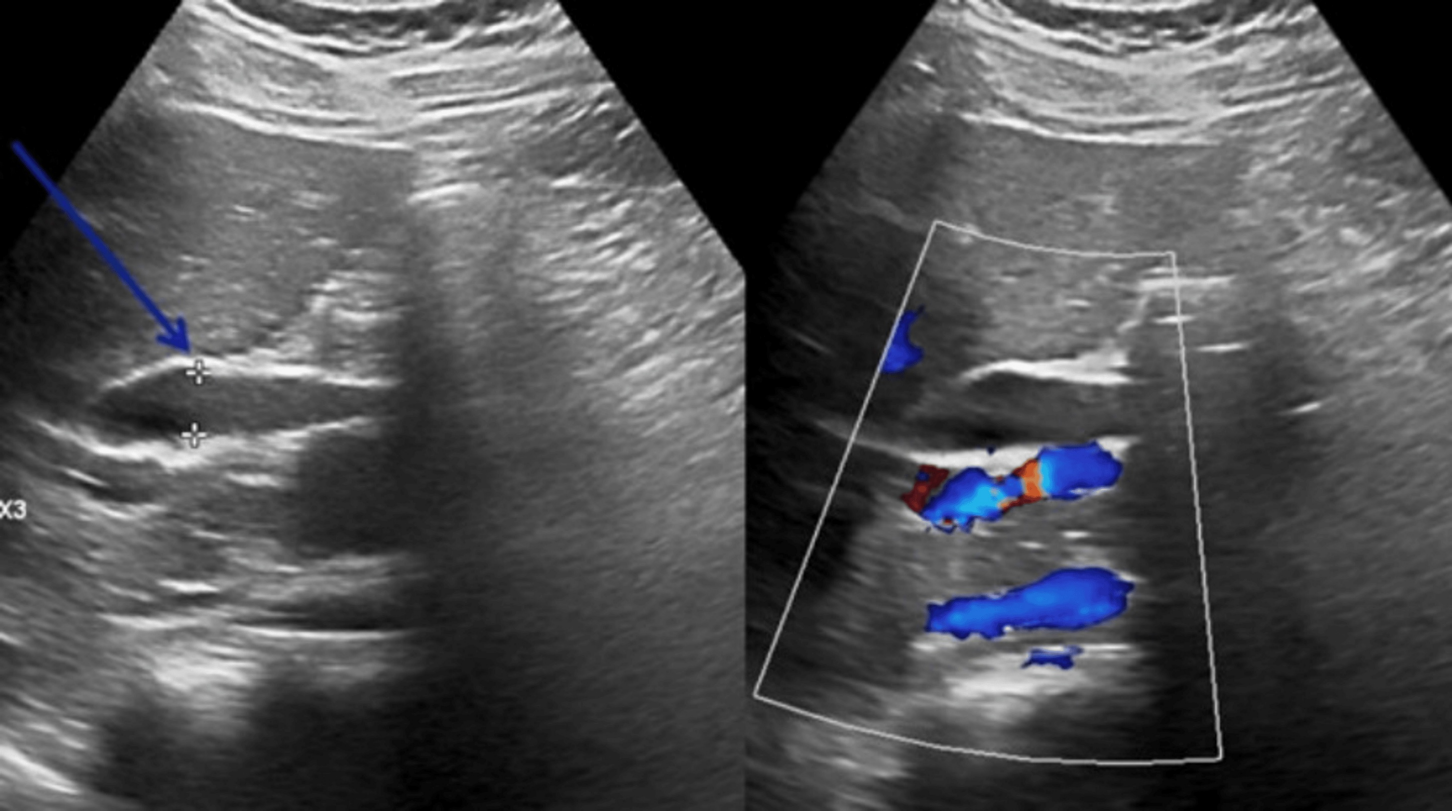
Abdominal ultrasound A transverse image demonstrating a dilated common bile duct, measuring 10 mm in diameter (indicated by calipers). This finding of biliary dilatation without evidence of choledocholithiasis prompted further cross-sectional imaging. The gallbladder and peripancreatic region are not well visualized in this image due to technical limitations and bowel gas interference.
